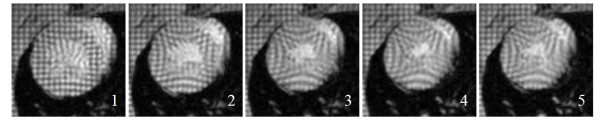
Tagged MRI images of the LV in the short axis view are shown for the systolic part of the cardiac cycle (*i *= 1,..,5). The myocardium exhibits relatively large displacements due to contraction.
